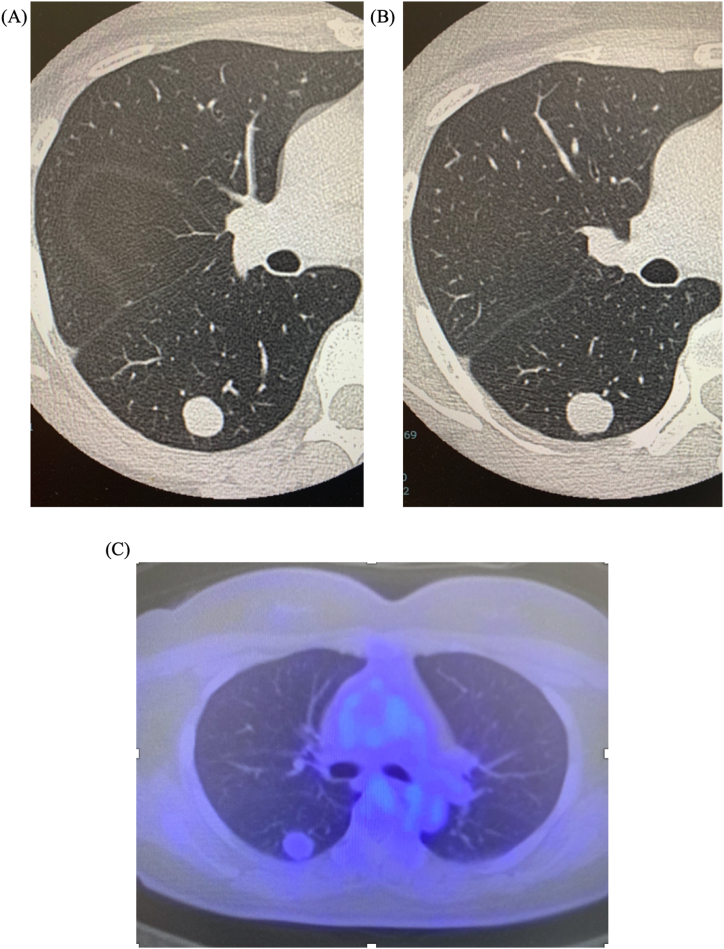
The preoperative image findings of the patient, a 46-year-old woman. (A) Computed tomography revealed a well demarcated 14-mm solid lung tumor in the right lower lobe (*arrowheads*). (B) A follow-up CT image ∼12 months later showed a slight increase in the tumor's size to 16 mm. (C) PET-CT indicated FDG accumulation with an SUV of 1.52 at the tumor, with no significant accumulation at any other site (*arrowheads*).
